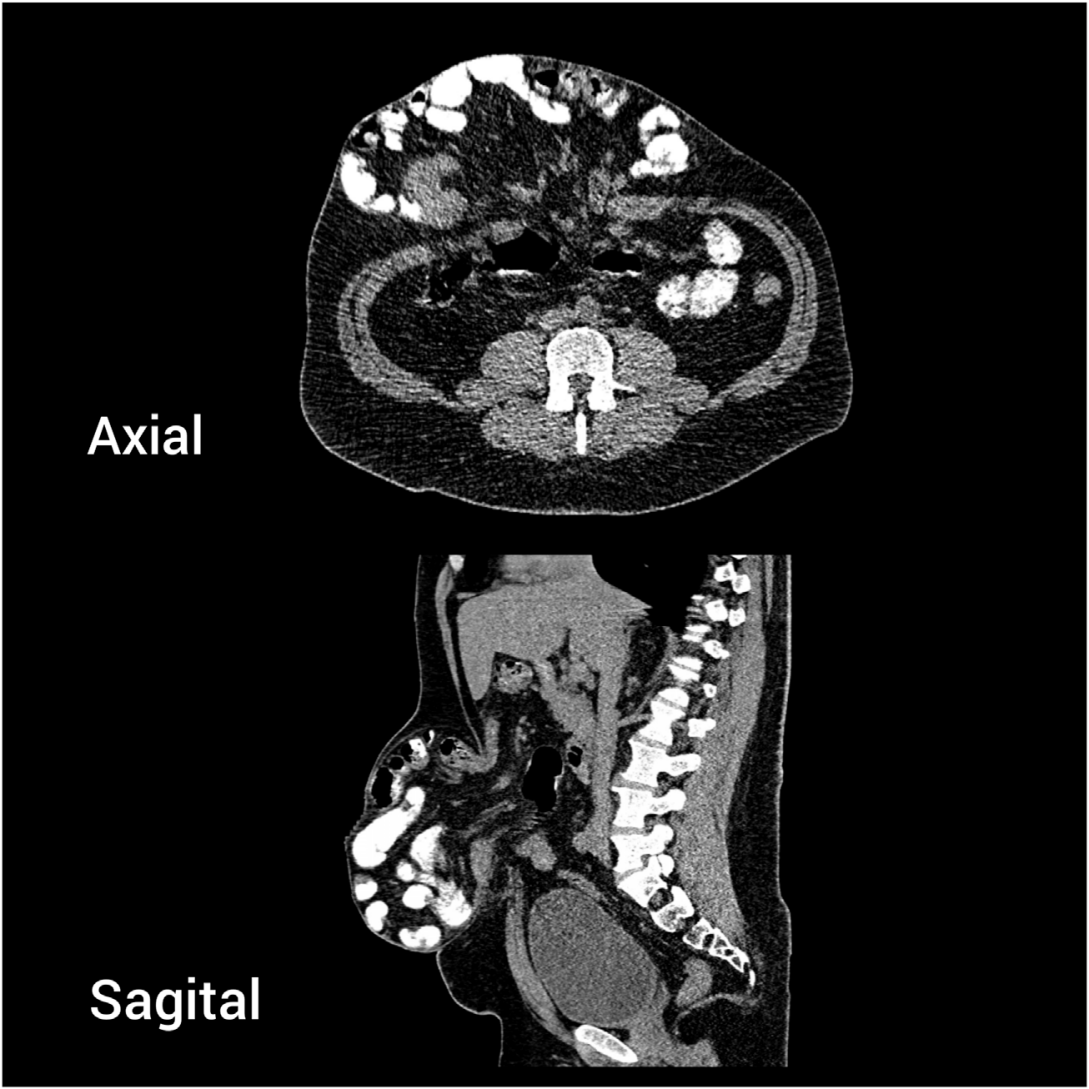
Case 1 - Preoperative CT scan. Axial and sagittal view.
